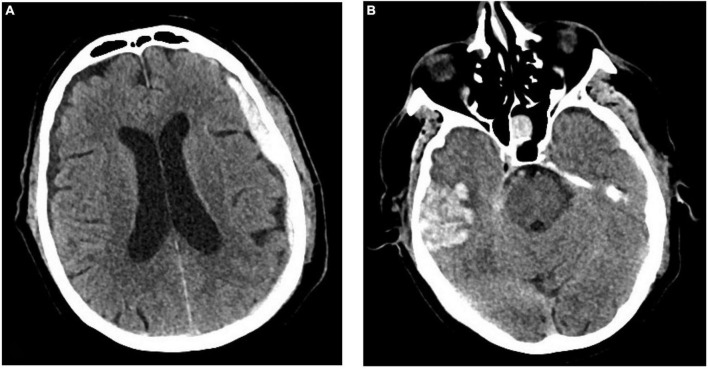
Exemplary cranial CT images of a 73-year-old male patient admitted to the emergency department of the University Hospital Frankfurt after a ground-level fall under ASA premedication. The CT scan shows the simultaneous presence of a subdural hematoma (A) and an intracerebral hemorrhage (B). ©Department of Diagnostic and Interventional Radiology, University Hospital Frankfurt, Frankfurt am Main, Germany.
